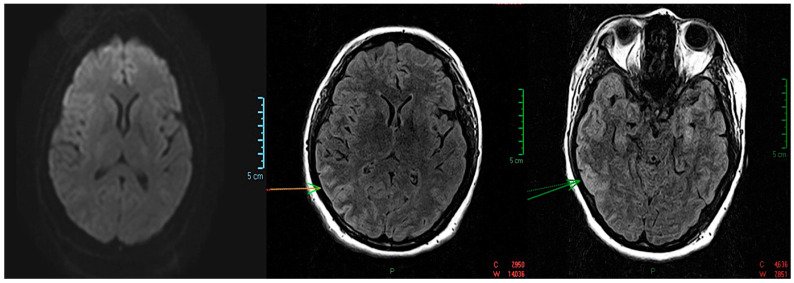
The initial native brain MRI revealed: axial hyperintensity on the T2 and FLAIR sequences of the right temporo-occipital cortex; a “Swollen” appearance of the right temporo-occipital cortex, in hyper signal T2 and FLAIR (arrow), without a correspondent on the diffusion or T2* sequences, associated with moderate erasure of the intergyral grooves but without a mass effect on the midline structures; the imaging appearance raises the suspicion of a temporal cortical edema—parietal; no other abnormalities of brain signal or morphology, and no lesions with diffusion restriction or endocranial bleeding scars; normally positioned and sized ventricular system.
